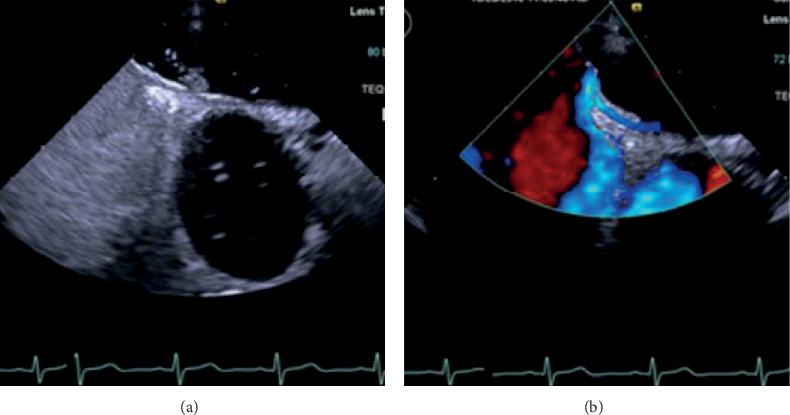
Contrast transesophageal echocardiographic (cTEE) images of PFO. PFO was detected with right-to-left shunting (a) or flow signal (b).
